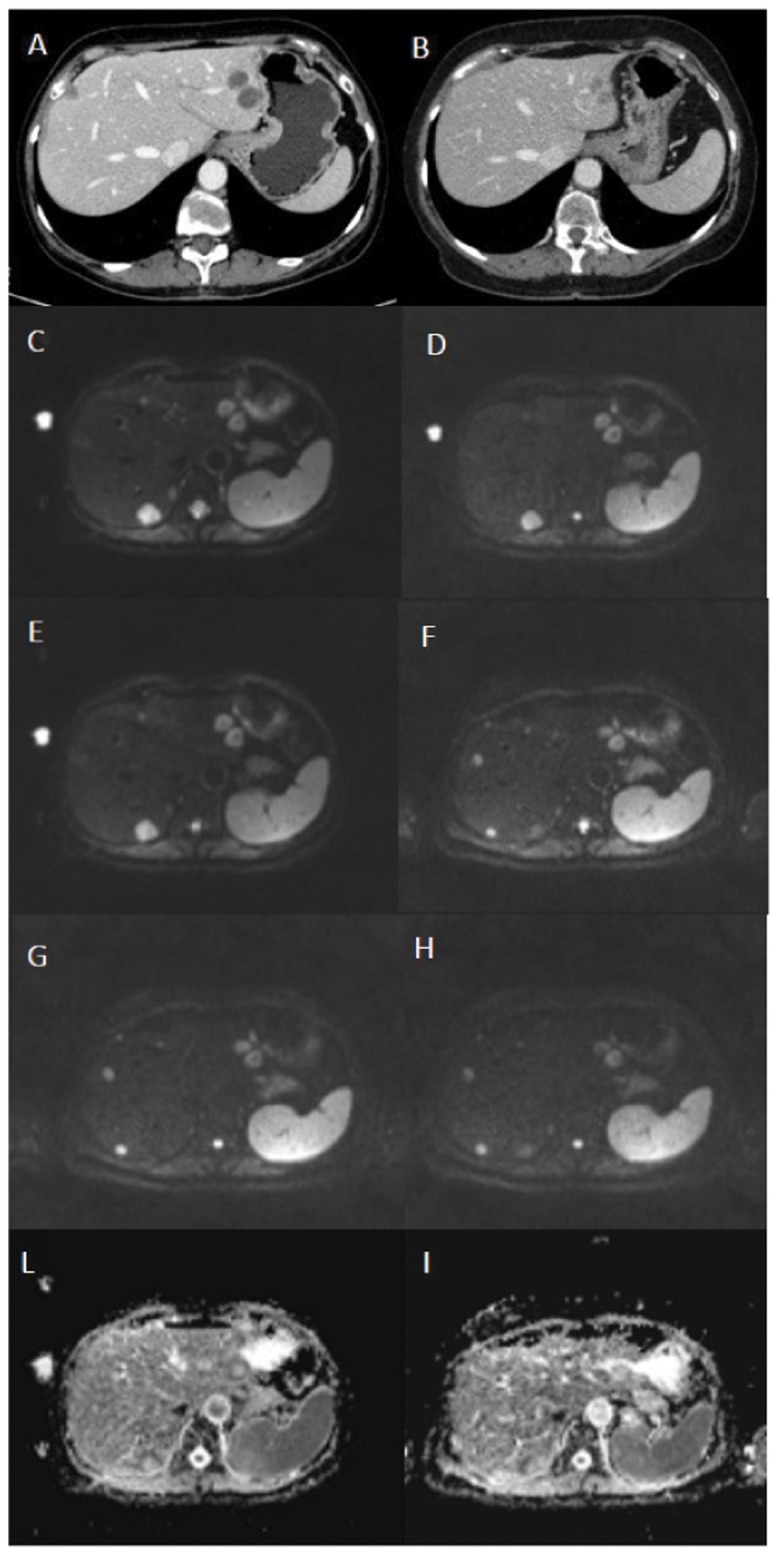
45-year-old woman with liver metastasis from colorectal cancer. Portal-phase axial CT scans before (A) and three months after (B) treatment show a decrease in size of two left liver lobe lesions and disappearance of the right liver lobe lesion. Axial DWI images before (C, b value 50, D, b value 400, E, b value 800) and 14 days after treatment (F, b value 50, G, b value 400, H, b value 800) do not demonstrate significant differences in the signal intensity of the two left liver lobe lesions with disappearance of the right liver lobe lesion. Axial tissue diffusion maps before (I) and 14 days after treatment (J) do not show significant differences in the signal intensity of the two left liver lobe lesions with disappearance of the right liver lobe lesion. This demonstrates that the qualitative assessment and the quantitative tissue diffusion-based assessment are not sufficient to evaluate tumor response.
